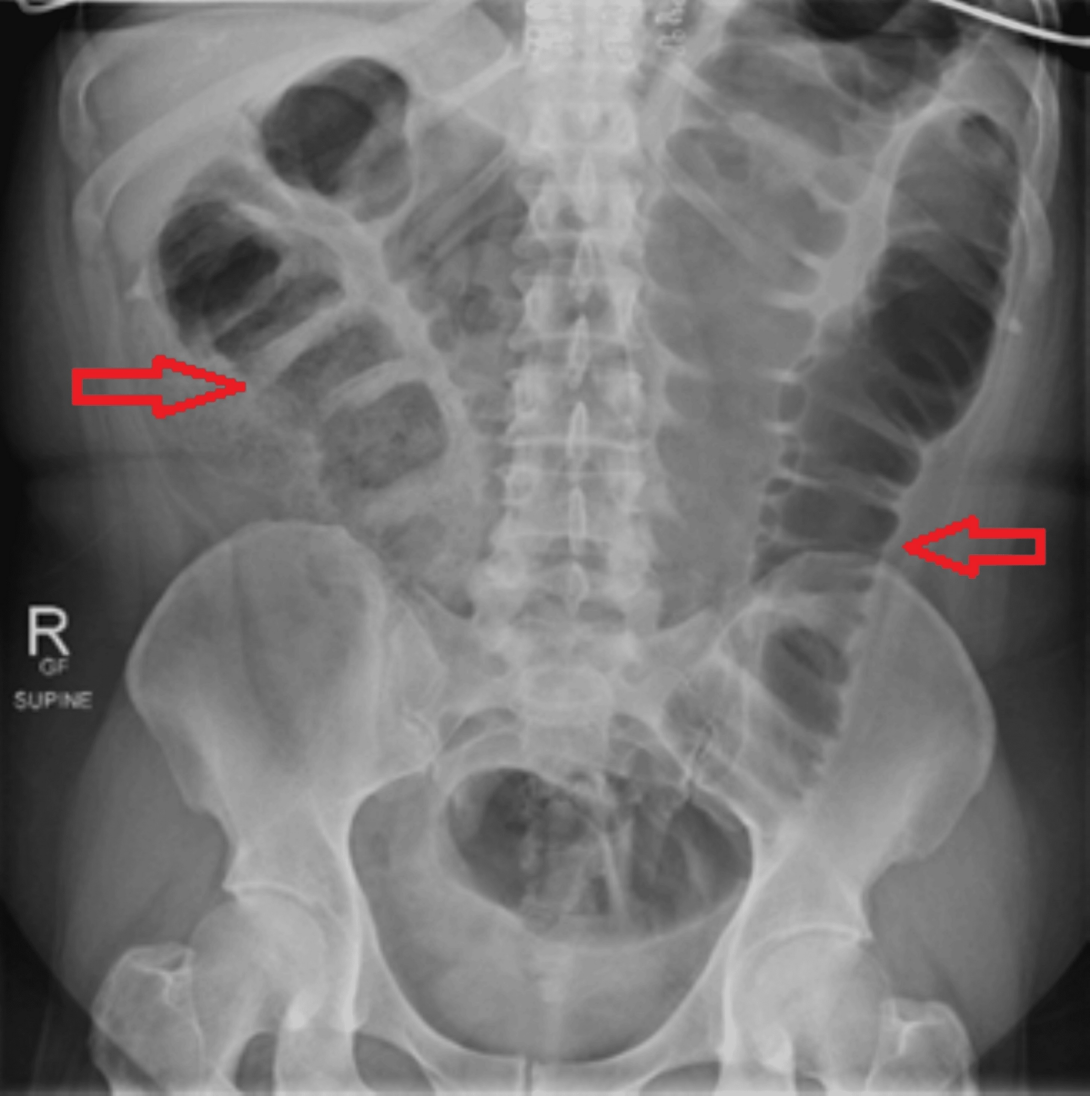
Abdomen X-ray showing distended large bowel loops (red arrows).
